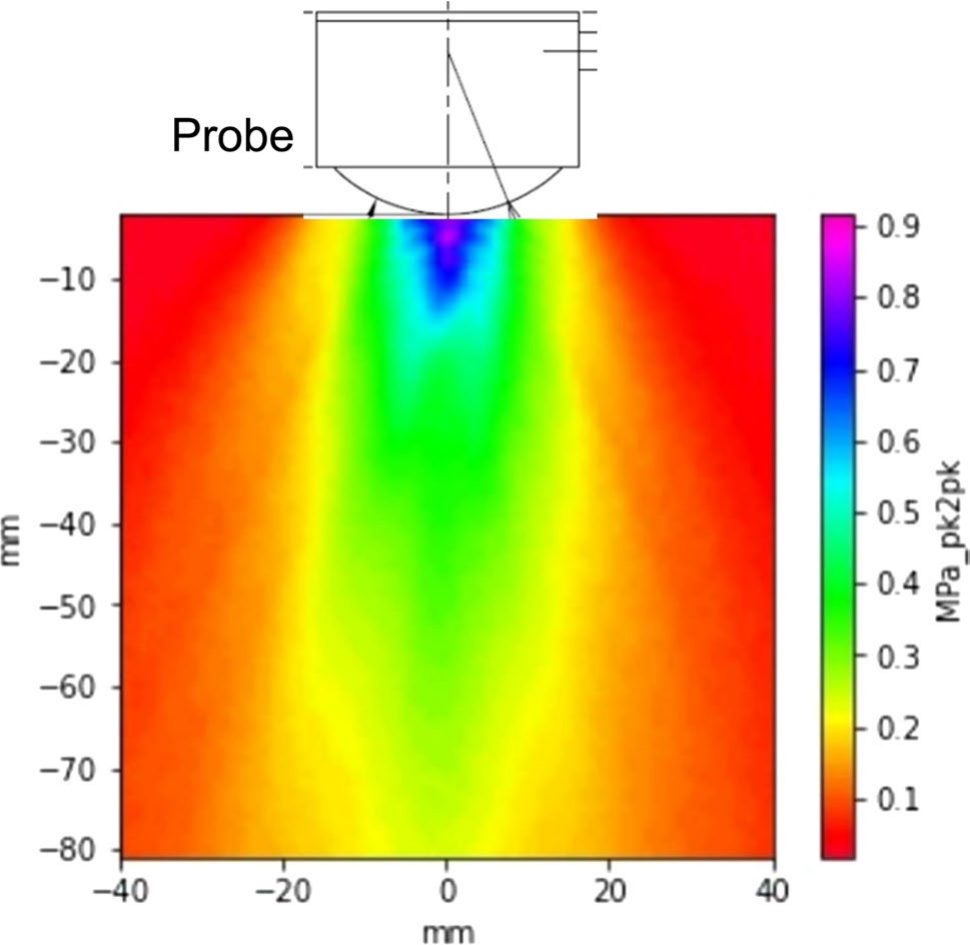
Sound pressure distribution of LIPUS therapy Sound field analysis of the ultrasound probe using the Acoustic Intensity Measurement System is shown. The energy just below the probe is the highest. We designed the ultrasound probe so that the sound pressure at a depth of 8 mm passing after the temporal bone would be within the therapeutic range (amplitude 0.5-1.0 MPa)
